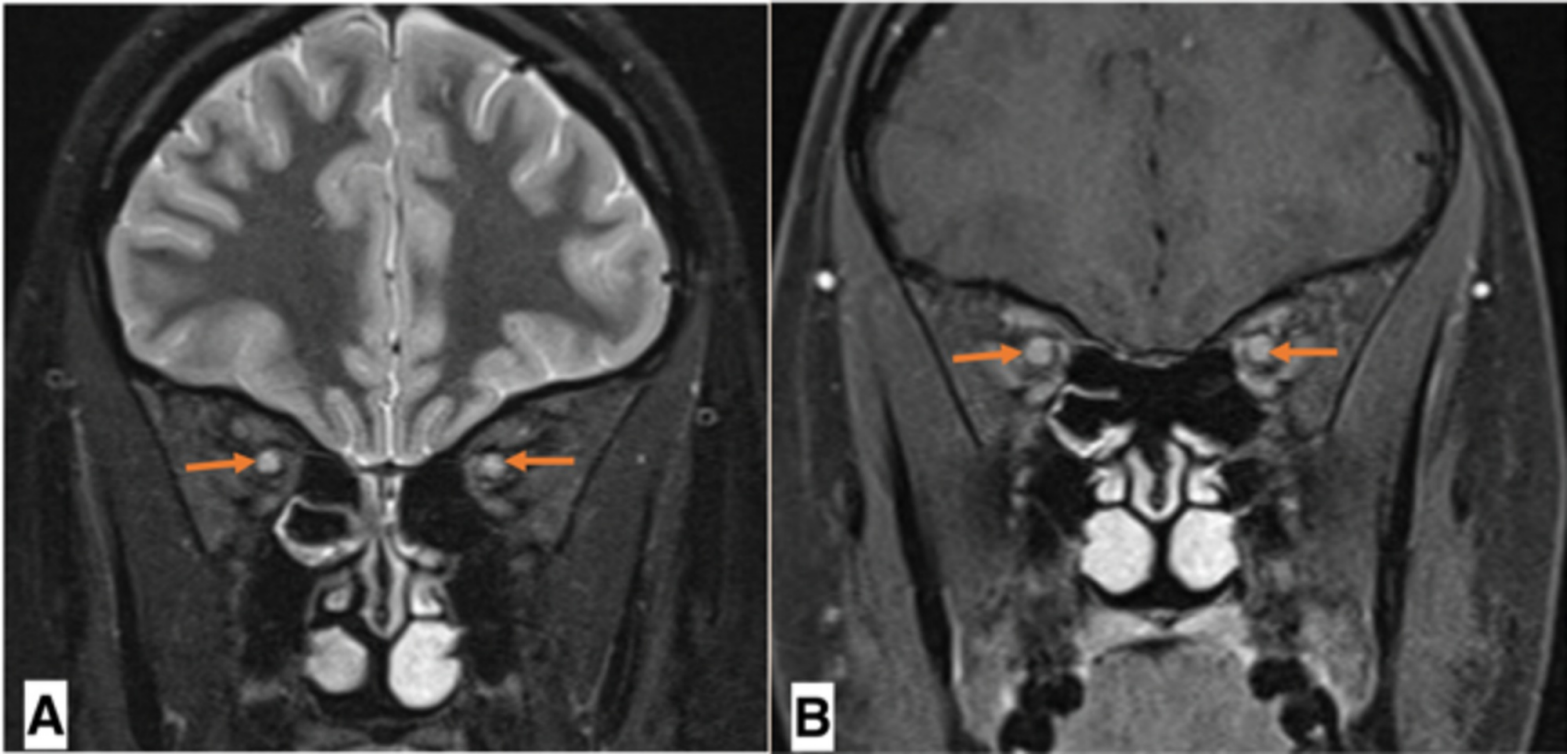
MRI of the orbit A: bilateral 'orbital' optic nerve segment (arrows) T2 prolongation and pathological enhancement. B: T2 prolongation and pathological enhancement of ‘prechiasmatic' segment of optic nerve (arrows) MRI: magnetic resonance imaging
